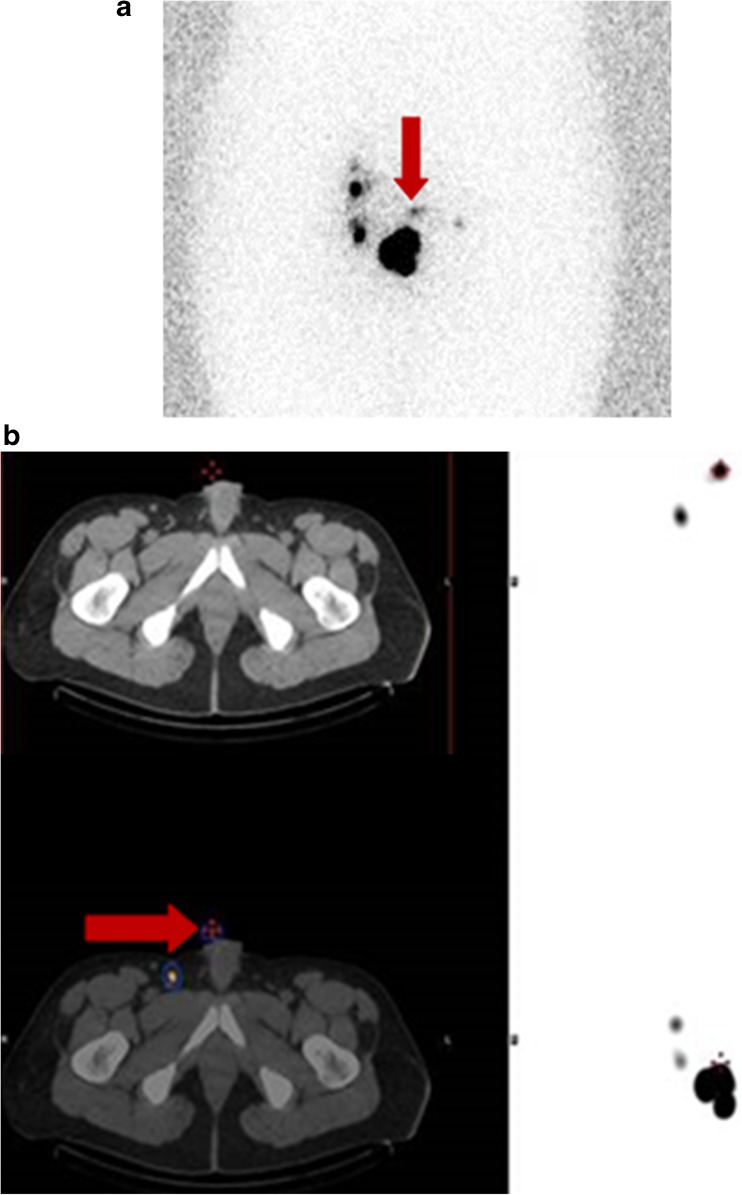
a Planar image showing tracer uptake (*red arrow*) resembling a lymph node in the suprapubic region. b SPECT/CT image demonstrates that the uptake noted in a actually represents contamination (*red arrow*)
